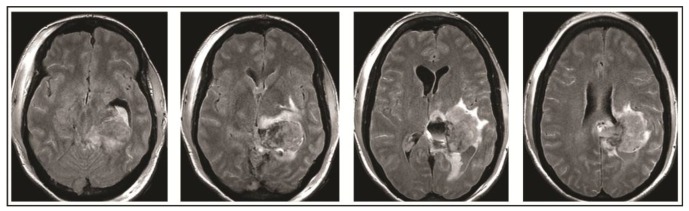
Post-operative day 1 MRI demonstrates 50% resection of left lateral ventricle meningioma.
